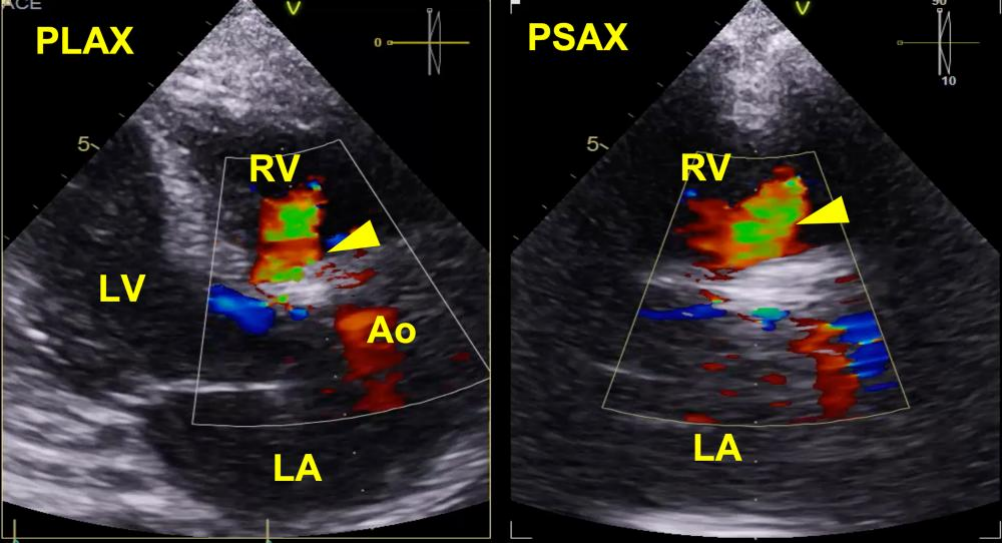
Transthoracic echocardiogram with colour flow Doppler evidencing an aortic-to-right ventricular fistula (arrowhead) status post-TAVR. Ao, aortic root; LA, left atrium; LV, left ventricle; PLAX, parasternal long axis; PSAX, parasternal short axis; RV, right ventricle.
